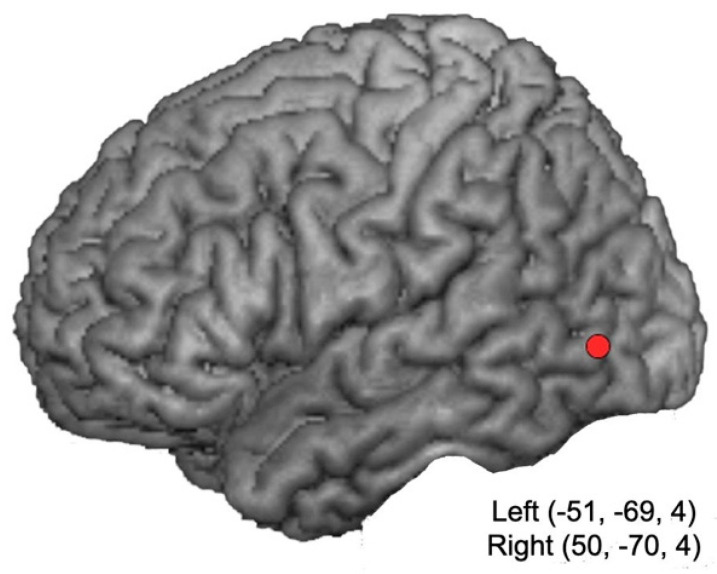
Stimulation sites marked on estimated MRI slices. The mean Talairach coordinates fell within the posterior part of the middle temporal gyrus (Brodmann’s area 37) for the left and right lateral occipitotemporal cortex.
